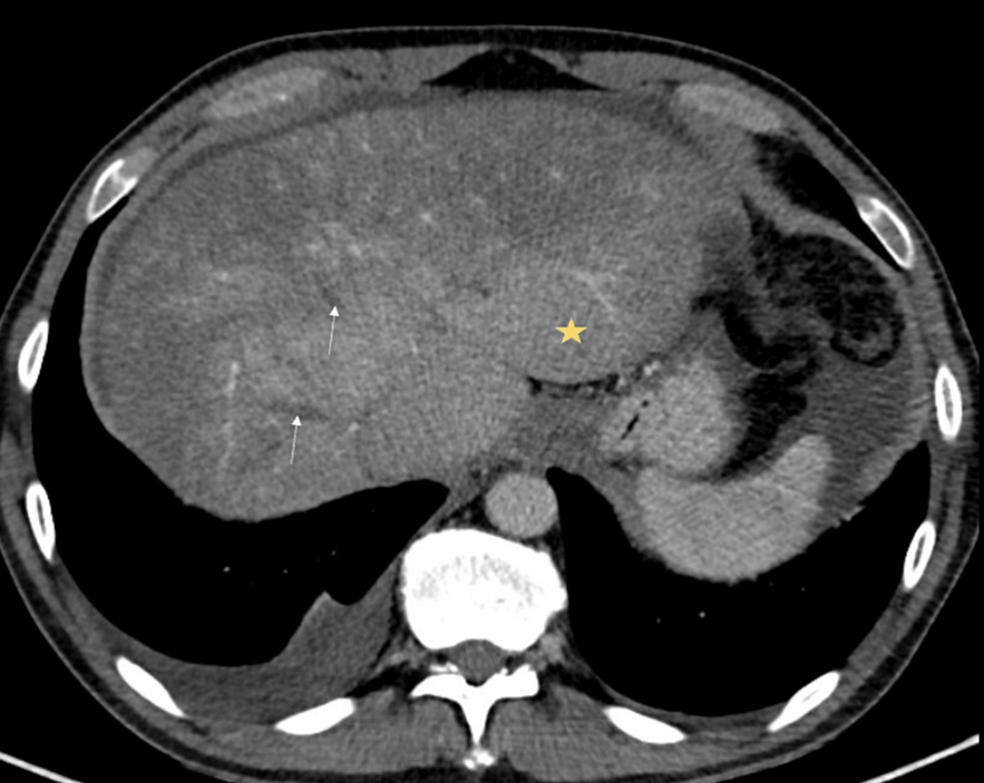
Contrast-enhanced abdominal CT in venous phase demonstrating findings suggestive of Budd-Chiari syndrome. The CT shows a heterogeneous pattern in the contrast uptake of the liver, with early uptake of the caudate lobe (marked with yellow star) associated with the absence of uptake of the suprahepatic veins (marked with white arrows).
